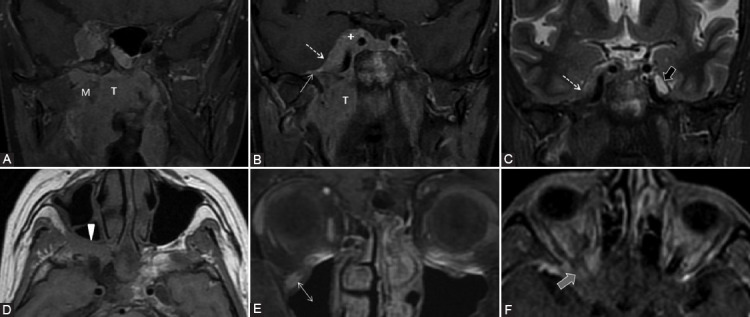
The right nasopharyngeal squamous cell carcinoma (T) in a patient with bilateral cervical adenopathy, right ptosis, and infraorbital skin paresthesia. Coronal contrast-enhanced T1-weighted fat saturated (A, B, E), coronal short-TI inversion recovery (C), axial T1 (D), and axial dynamic contrast-enhanced (F) images. There is tumor extension in the right masticator space (M) in the mandibular nerve distribution area, thus tumor cells spread retrograde to the foramen ovale (CN V3) which is enlarged (white arrow). Afterward, the neoplastic extension affects Meckel’s cave (dashed arrow) and cavernous sinus (+), with enlargement and CE. Notice the CSF signal in the normal left Meckel’s cave (black arrow) and it’s effacement on the right. Obliteration of the fat in the right PPF indicates CN V2 involvement (arrowhead). From here, there is anterograde PNS to the infraorbital nerve (double arrow). The right CN II invasion is also detected (gray arrow).
